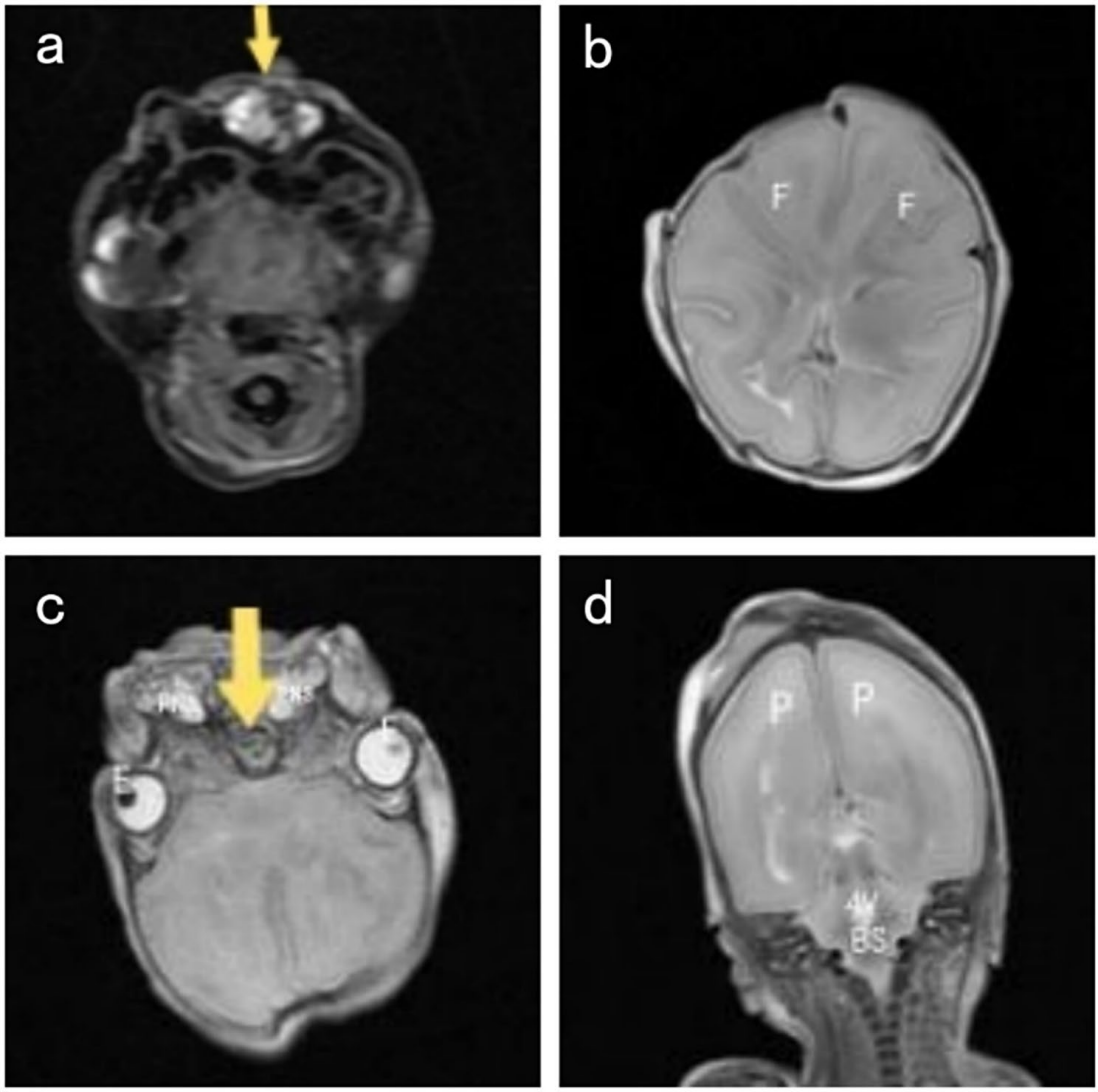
Postnatal MRI images of this case. (a) T1-weighted imaging reveals fusion of the bilateral maxillofacial bones as indicated by the arrow. (b) MRI T2-weighted imaging shows bilateral enlargement of the frontal lobes, widening of brain gyri, and an increased angle between the anterior edges of the lateral ventricles (F: Frontal Lobe). (c) T2-weighted imaging shows widening of the cranial bones in the frontal region, absence of eyeballs in the fused central eye region as indicated by the arrow, and two paranasal sinuses (E: Eye; PNS: Paranasal Sinuses). (d) T2-weighted imaging displays normal symmetric frontal and parietal lobes, brainstem, and fourth ventricle. (BS: Brain Stem; P: Parietal Lobe; 4 V: Fourth Ventricle)
